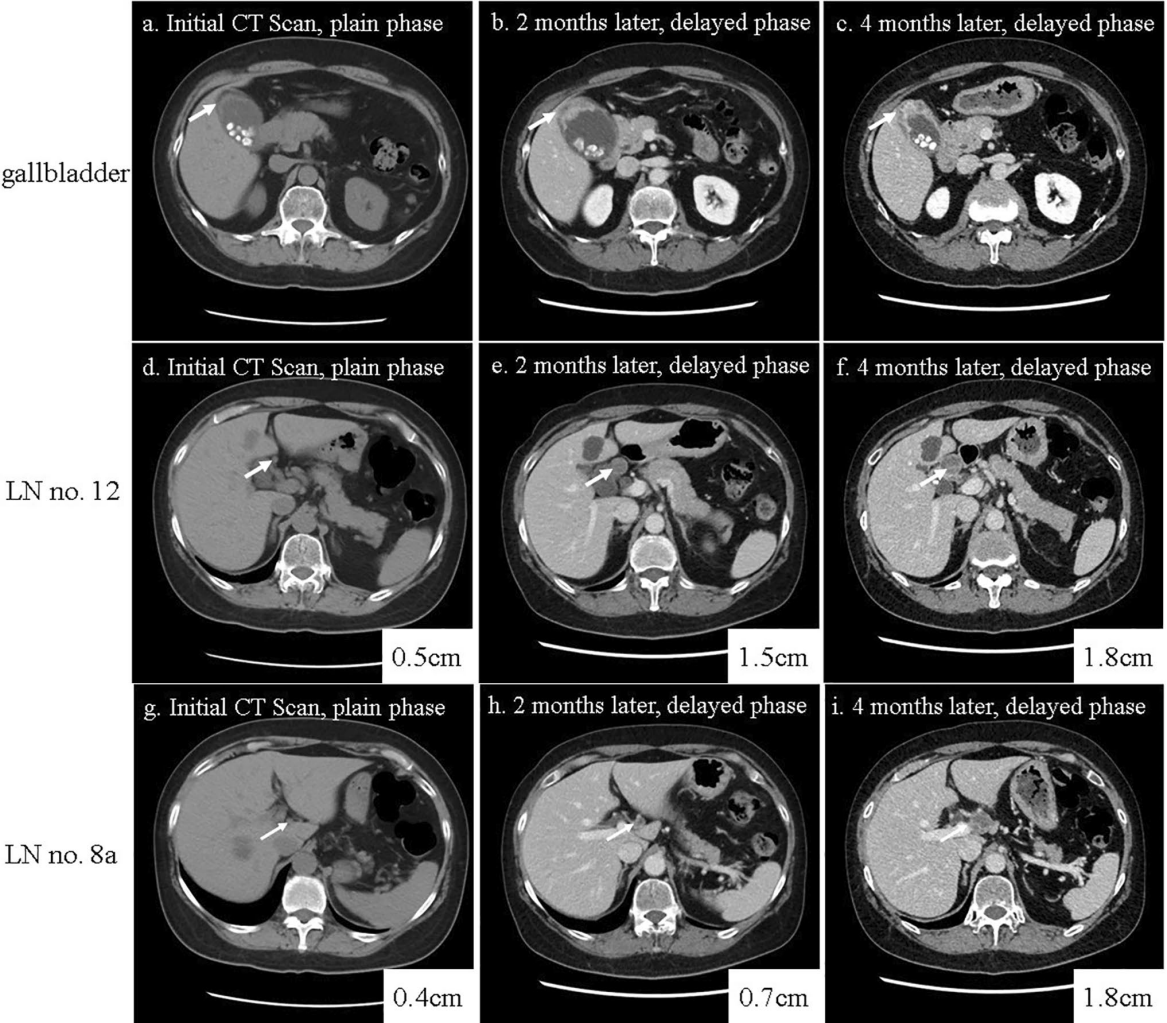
Preoperative serial CT scans of the gallbladder. The figure shows preoperative serial CT scans showing rapid tumor growth in the gallbladder and the adjacent LNs, over the course of 4 months. a, d, g CT scan at the time of surgical consultation. b, e, h Follow-up CT scan, 2 months later. c, f, i Follow-up CT scan, 4 months later. Initial CT scan showed no apparent tumors in the gallbladder or the LNs (a, d, g). CT scan at 2 and 4 months revealed gradual thickening of the fundus wall of the gallbladder (b, c), rapid growth of the LN no. 12 (e, f), and the LN no. 8a (h, i). No apparent liver infiltration or peritoneal dissemination were observed
